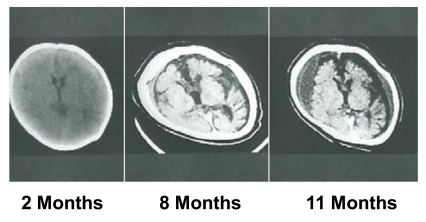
Brain CT images of a patient with Menkes disease at 2, 8, and 11
